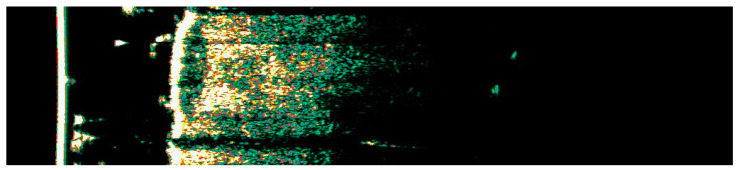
Ultrasonographic image of BCC on the arm.
